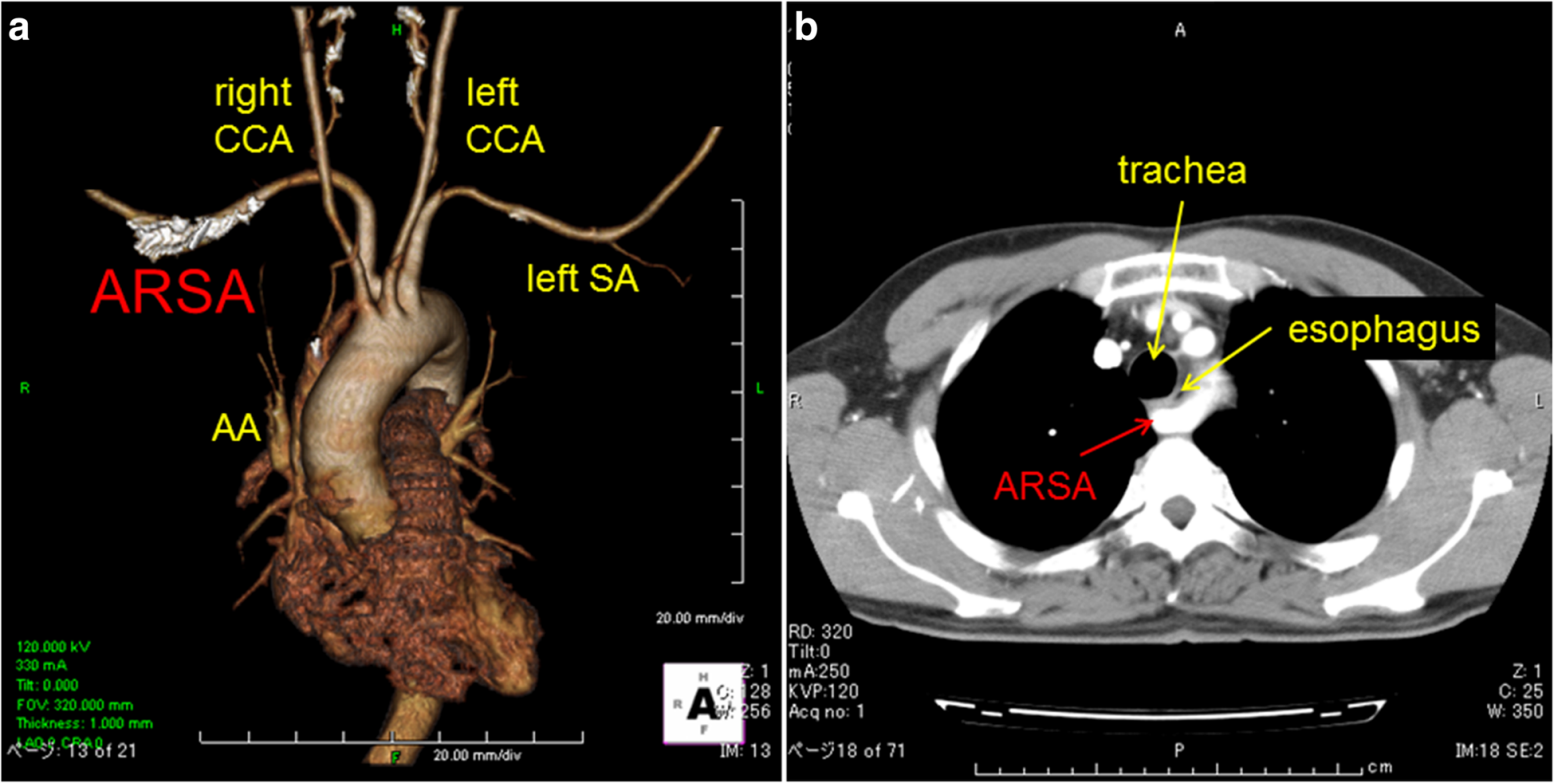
Case 1. a Three-dimensional and b enhanced computed tomography scan showed an aberrant right subclavian artery arising from the dorsal side of the distal descending aorta and passing through the retroesophageal space. *AA* aortic arch, *SA* subclavian artery, *CCA* common carotid artery
